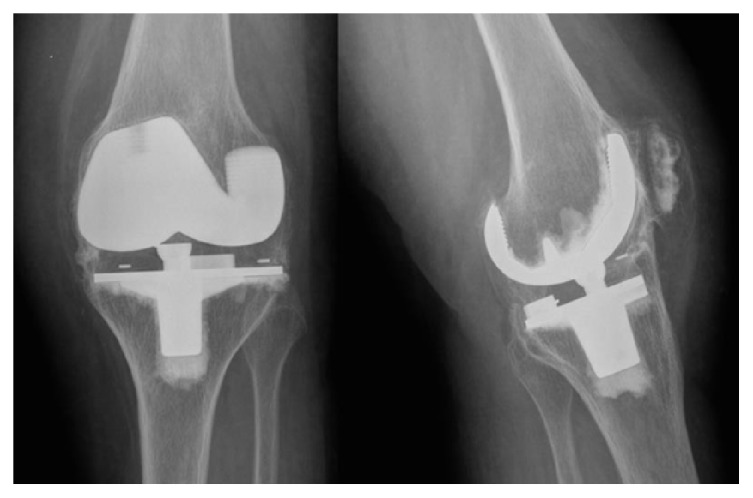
AP and lateral X-rays showing massive heterotopic ossification around the implants.
